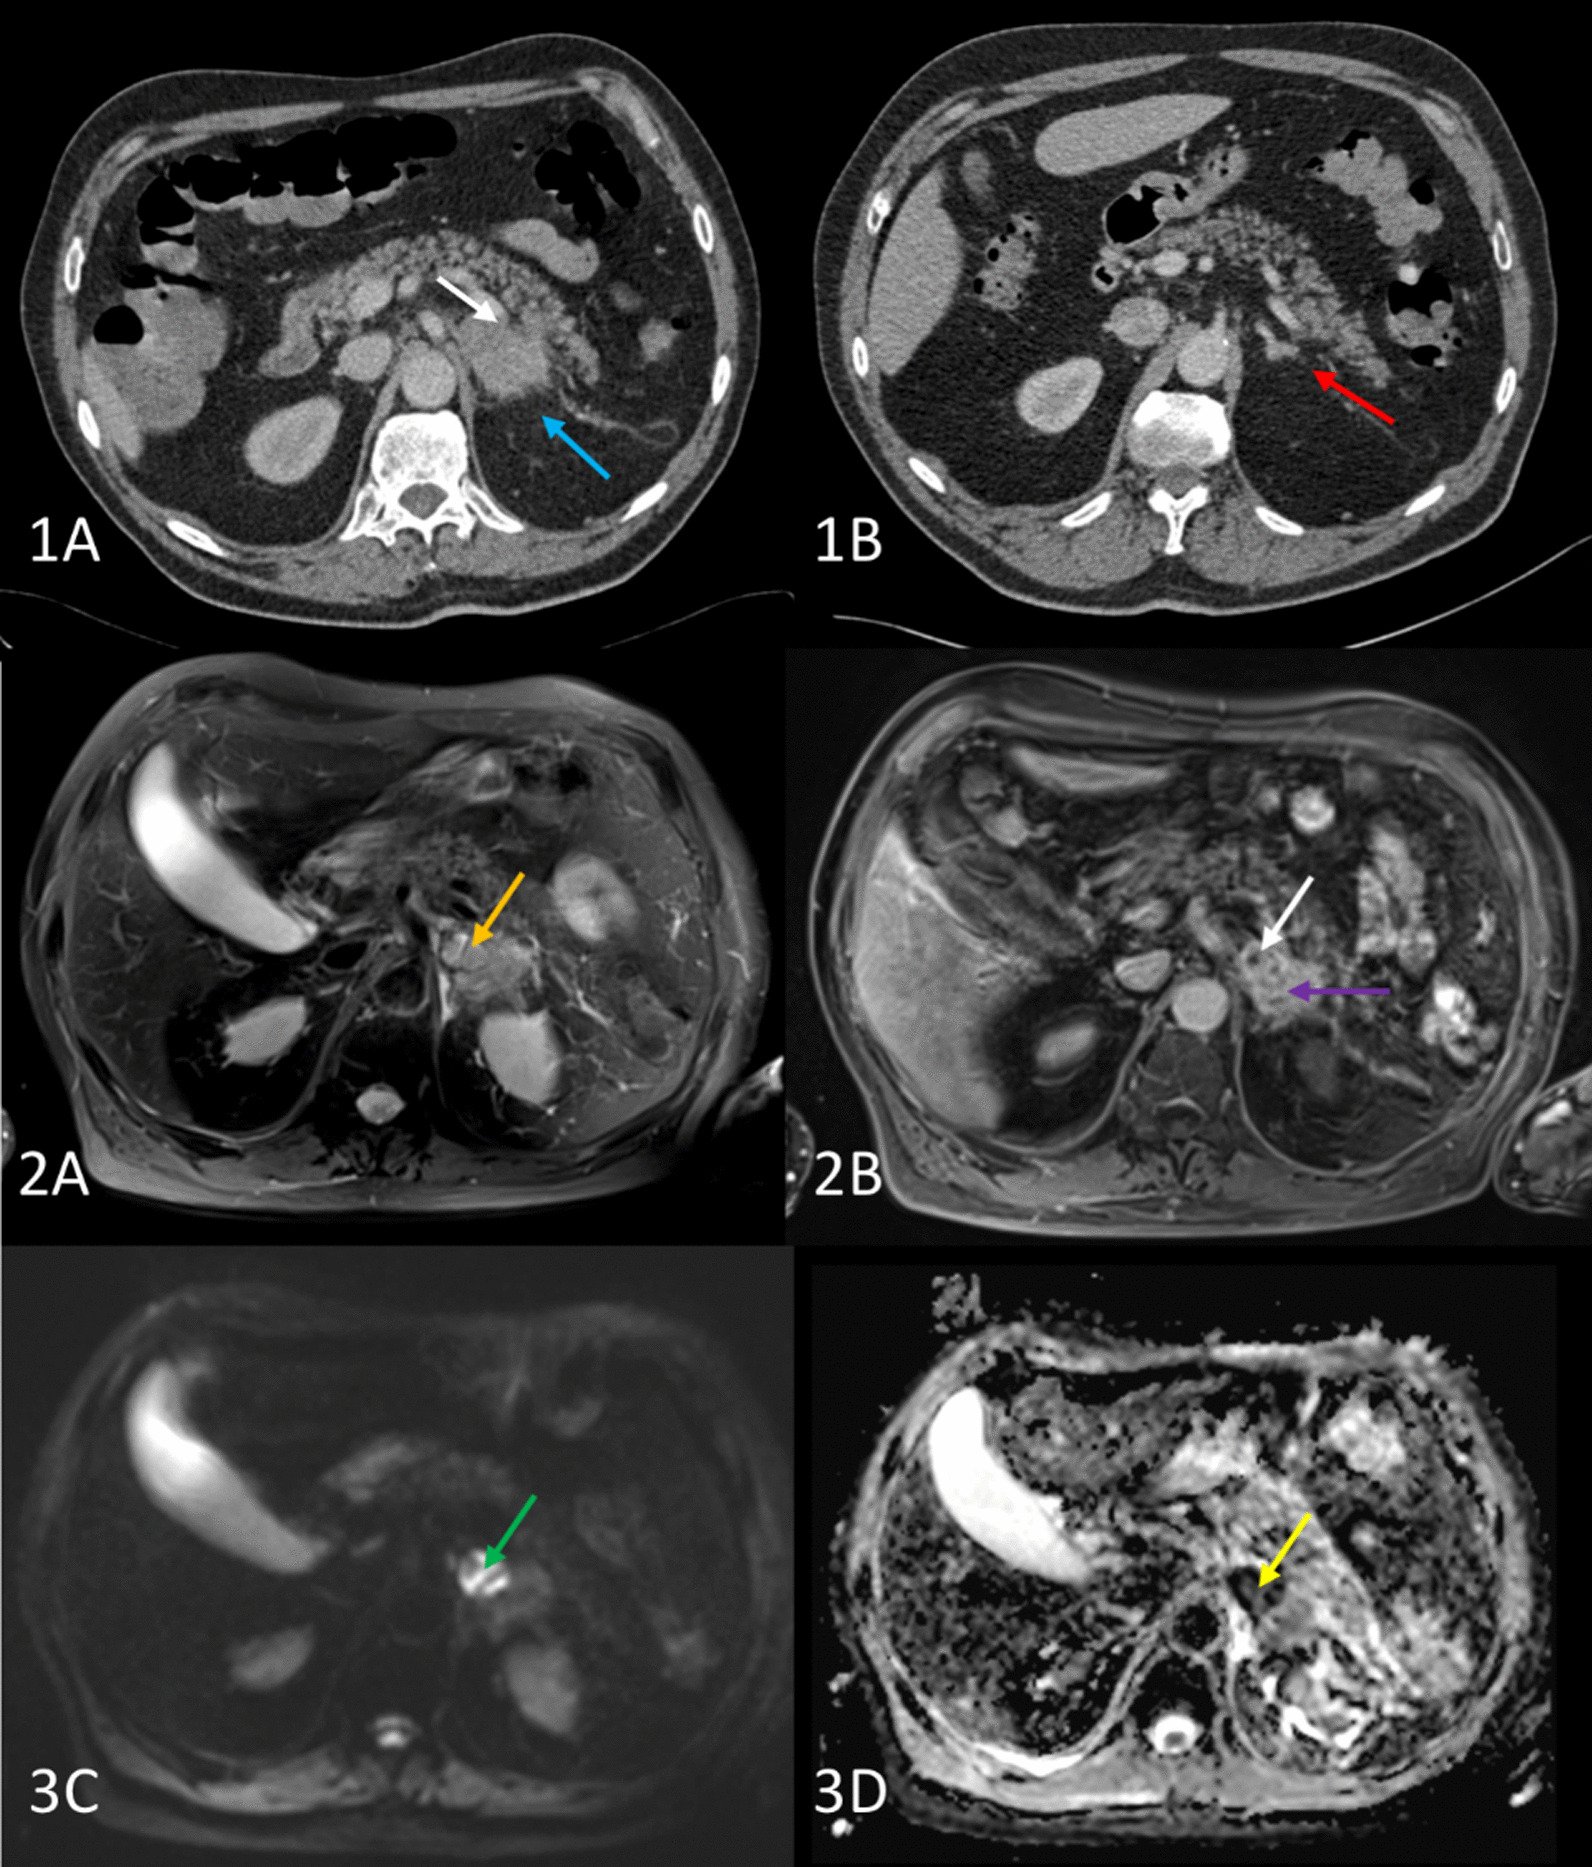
Abdominal CT and MR scan. Abdominal CT scan [1] in axial view, initial (1A) and 6 months after starting antibiotic treatment (1B). The large left adrenal abscess (white arrow) is accompanied by inflammation of the surrounding fat (blue arrow). Note the normalization of the left adrenal gland (red arrow) and the disappearance of the inflammation of the surrounding fat. MR images [2] acquired during the antibiotic treatment. The T2 with fat saturation images (2A) shows a hypersignal in the anterior part of the left adrenal gland (orange arrow). On the enhanced T1 sequence (2B), this image is not enhanced (white arrow) corresponding to the central part of the abscess (necrosis), while the rest of the adrenal gland is normally enhanced (purple arrow). The hypersignal in diffusion sequence (3C, green arrow), especially with a very low ADC (apparent diffusion coefficient) (3D), here less than 0.5·10^− 6^mm^2^ s^− 1^ (yellow arrow), leading to the diagnosis of abscess
